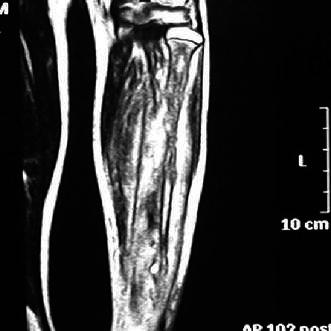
MRI of fibula with signal abnormalities in medulla, metaphysis, and diaphysis
